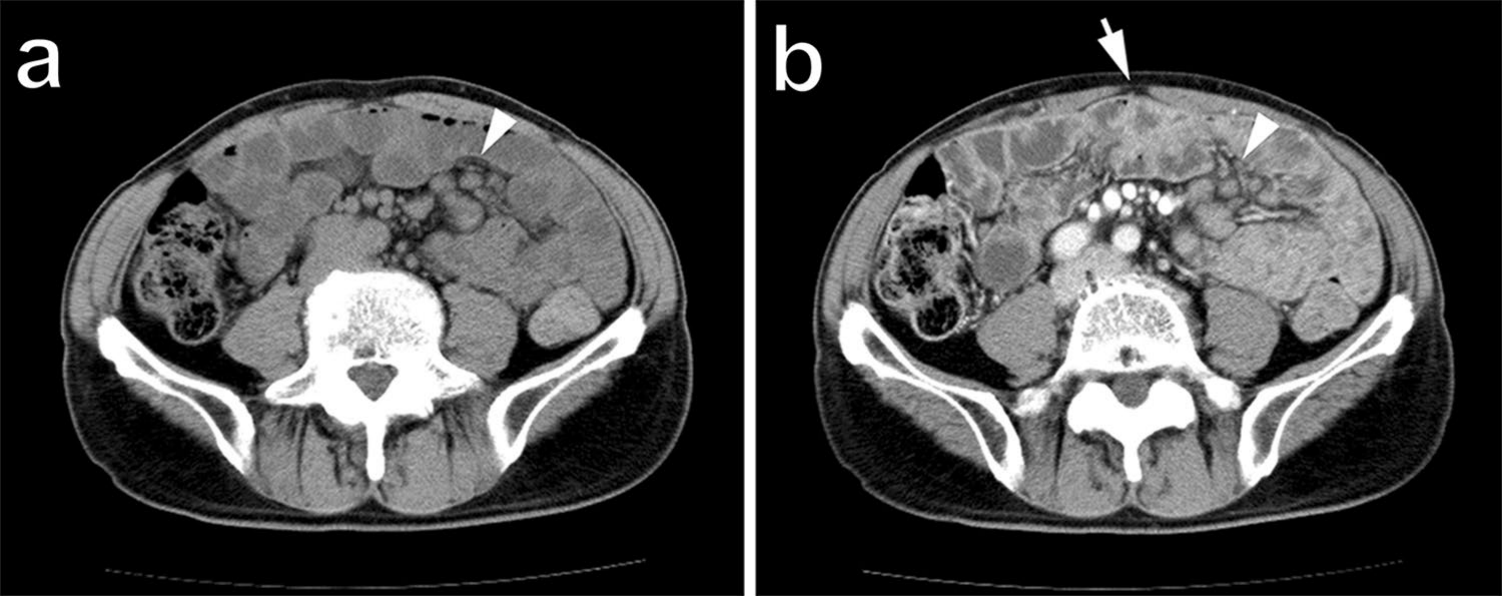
CT of pelvis without (a) and with contrast (b). Arrow and arrowhead point to the thickened wall of small intestine and the swollen lymph nodes, respectively
